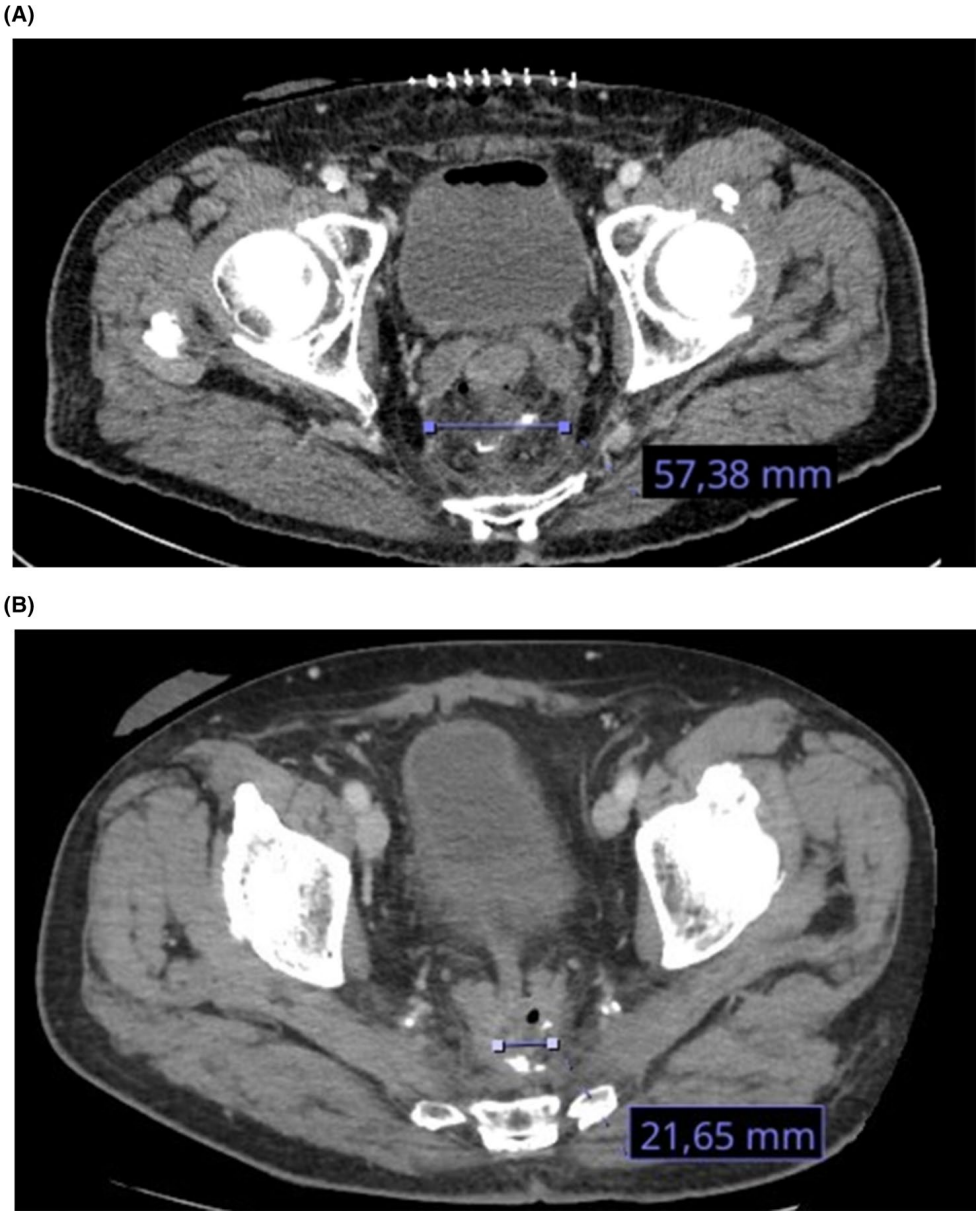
(A) Patient 10, initial CT scan on postoperative day five after low anterior resection of the rectum, showing a seroma of 57 mm. Culture of the collection fluid grew
